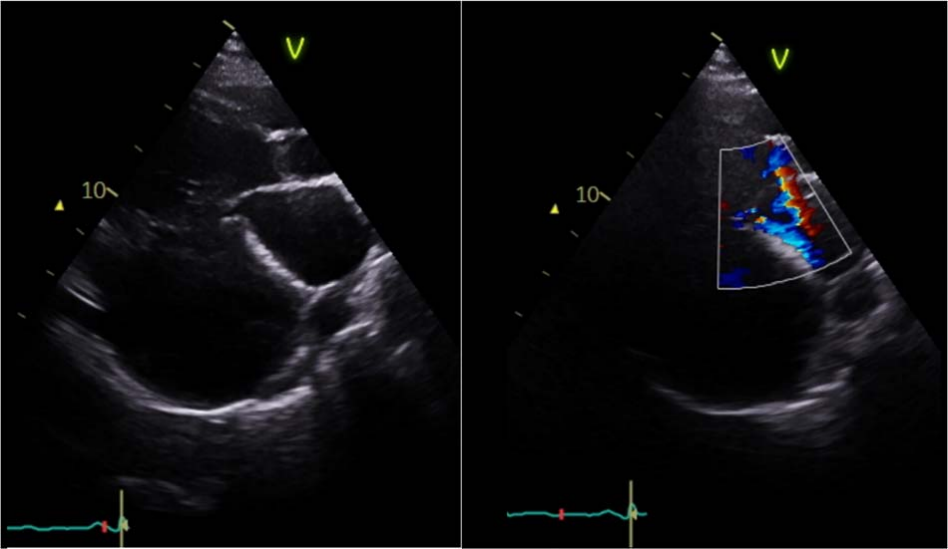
Postoperative echocardiography: restoration of mitral valve leaflet coaptation and LV geometry, resulting in trivial regurgitant jet.
